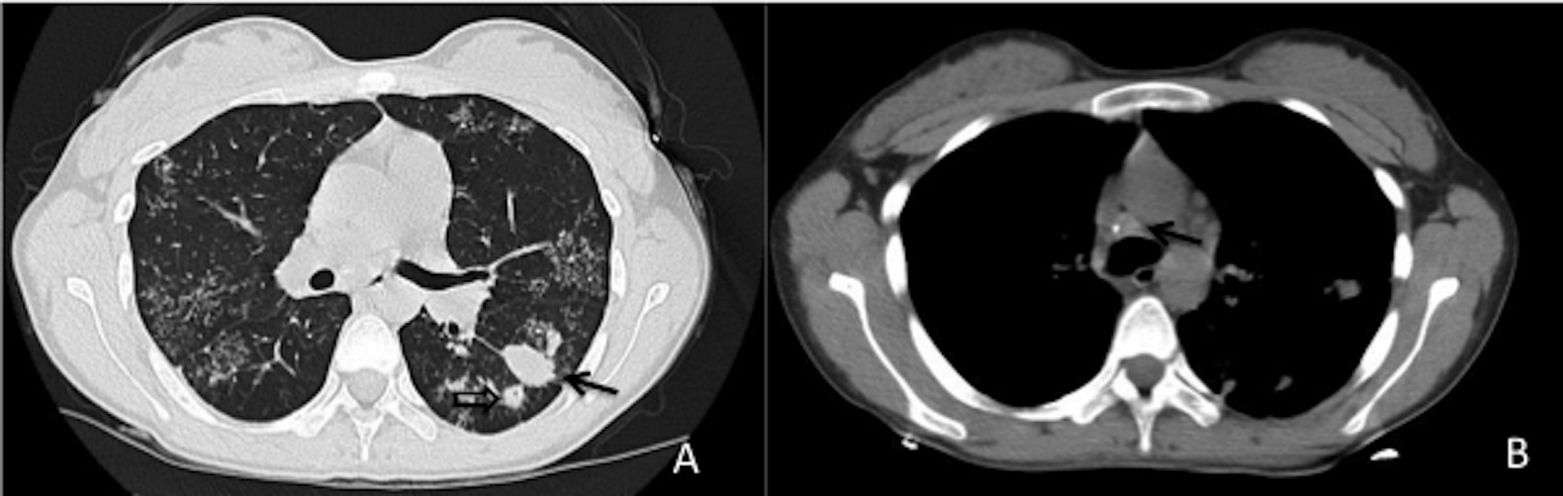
Drug-sensitive TB in a 20-year-old woman. A. Lung window of a transverse thin-section CT scan (1.25-mm-section thickness) showing a large nodule (arrow) and small nodules (empty arrow) in the left lower lobe. Tree-in-buds were also observed on both sides. B. Mediastinum windows of a transverse CT scan (5.0-mm section thickness) showing an enlarged lymph node with calcification (arrow).
